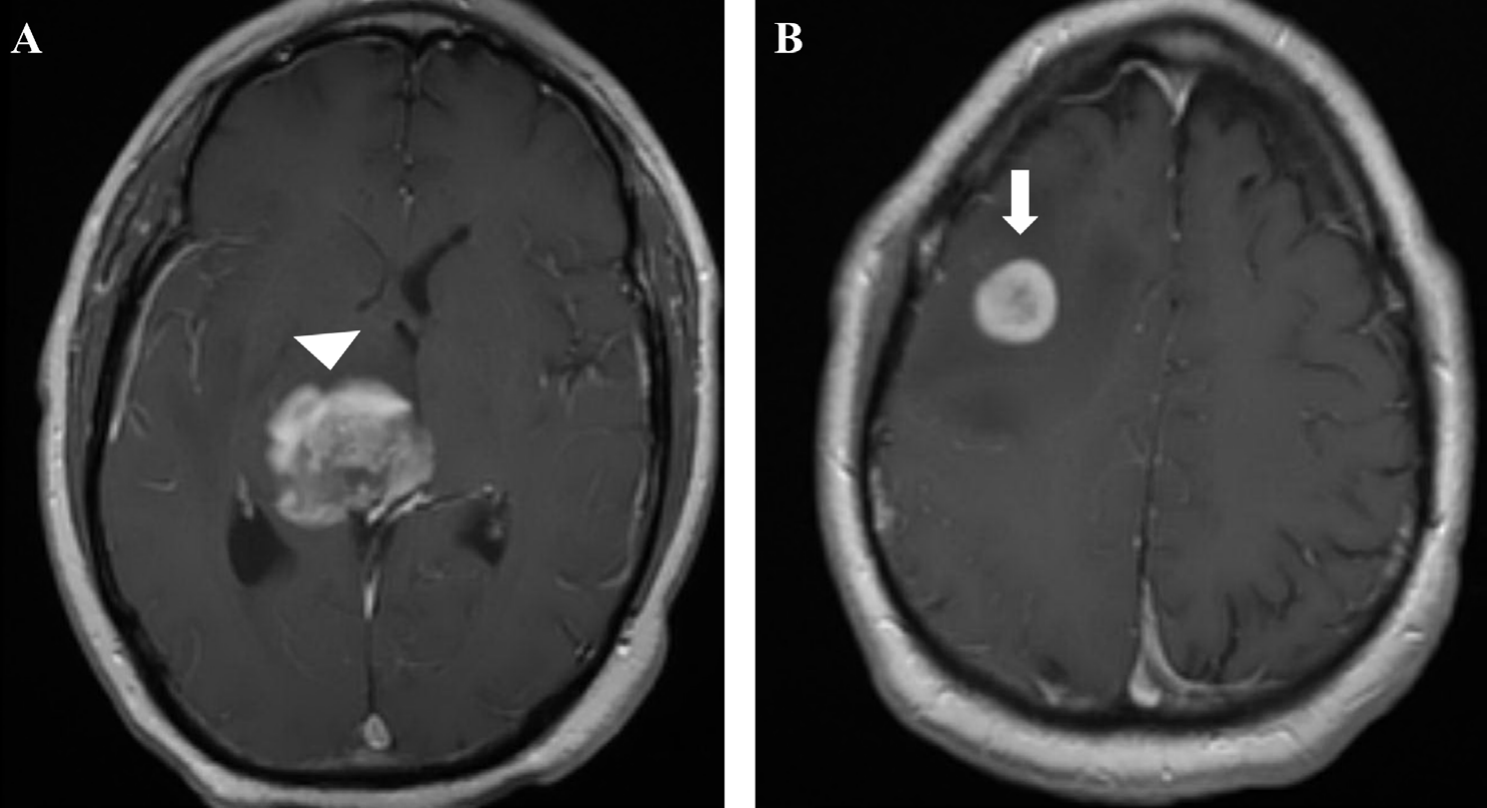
Follow-up imaging at 2 months after completing chemoradiation therapy (4 months since initial presentation). Axial contrast enhanced T1W MRI showing further growth of the primary tumor (white arrowhead), (A) and secondary tumor (white arrow), (B) along the biopsy tract.
